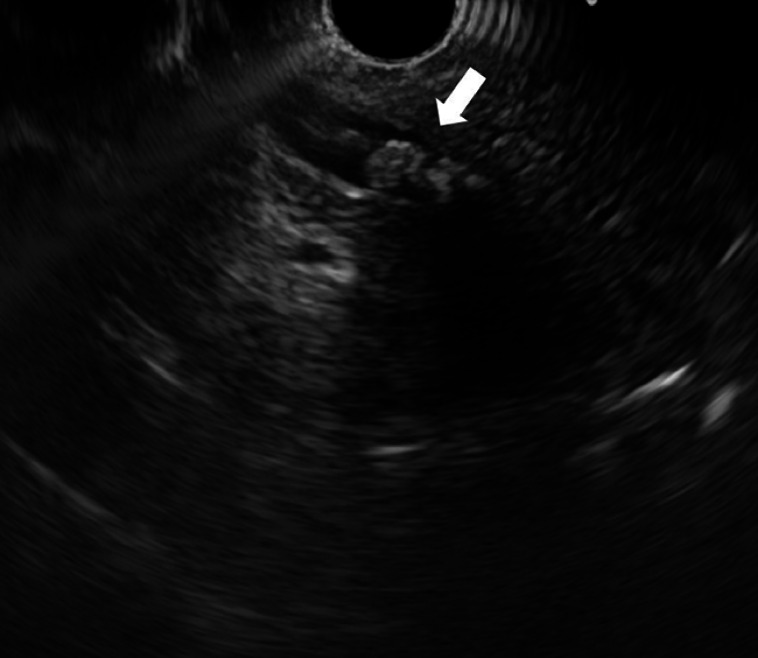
Endoscopic ultrasonography showing common bile duct stones.
